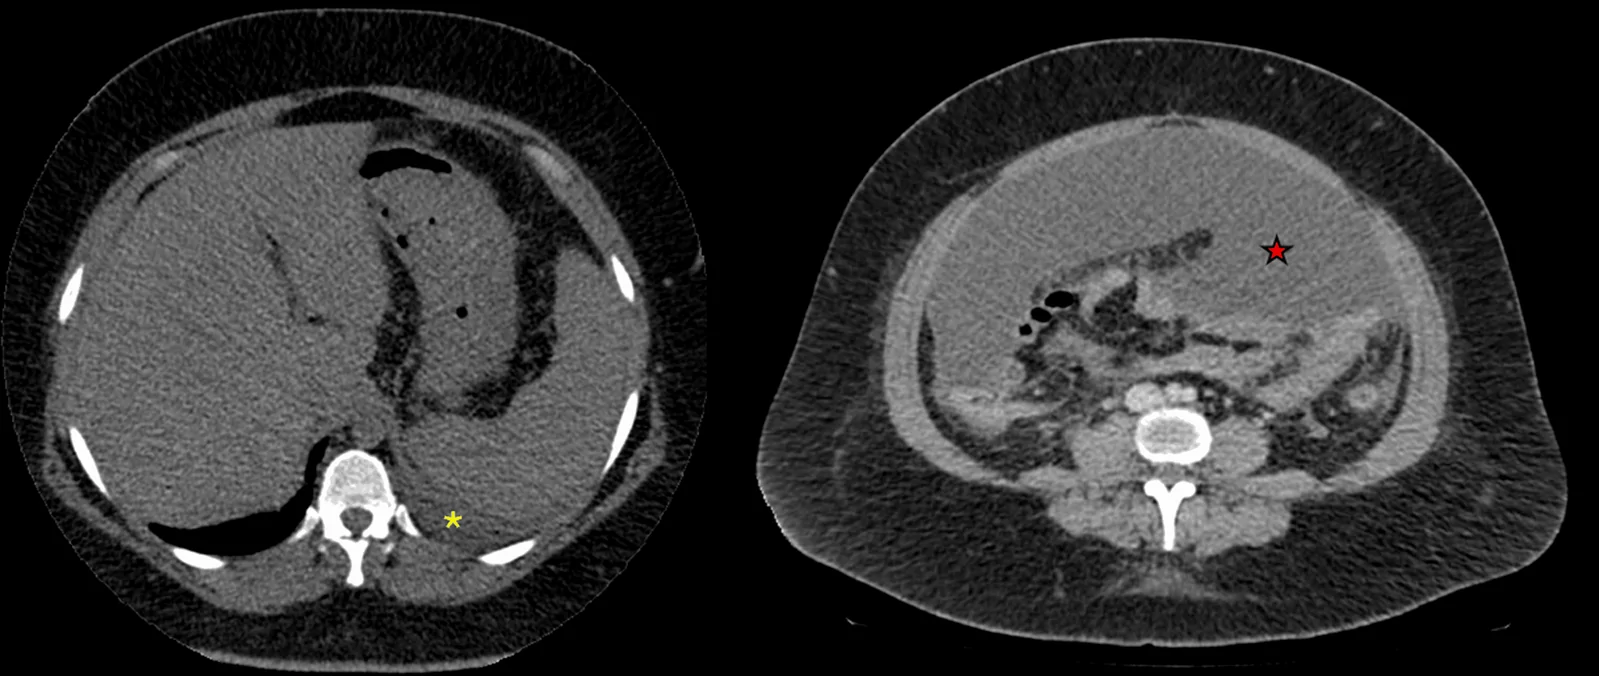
Pre- and post-contrast abdominal CT scan (axial view) Small left pleural effusion (asterisk) and ascites with atypical distribution in the inframesocolic intraperitoneal compartment (red star). CT: computed tomography
